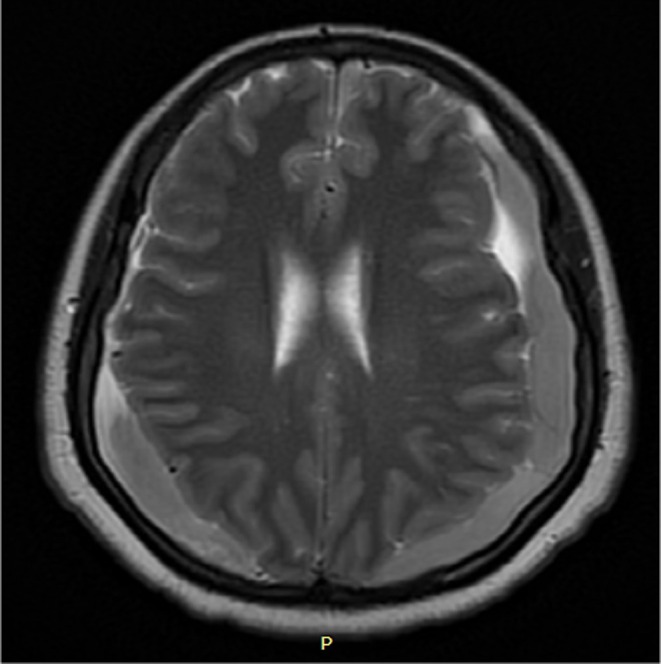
A MRI image showing right side acute subdural hemorrhage in the parietooccipital area and left hemispheric acute subdural hemorrhage.
